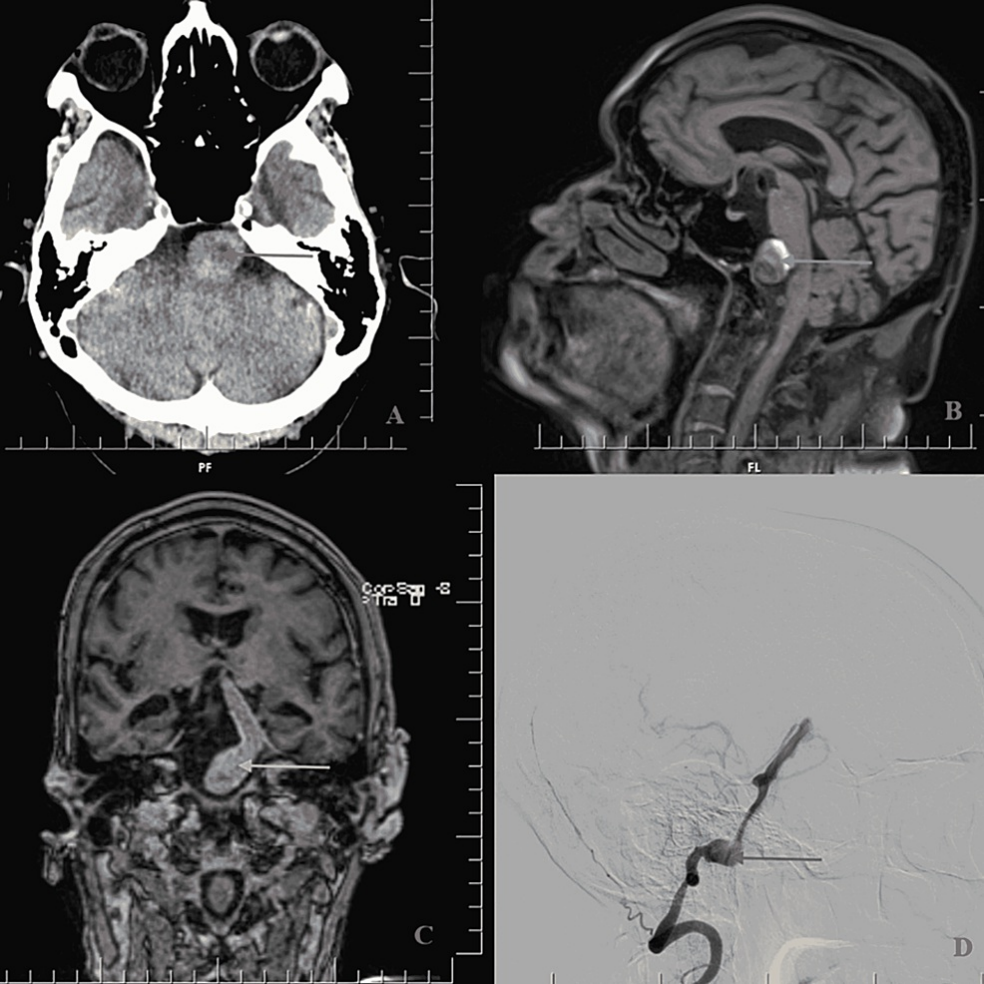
(A) CT brain axial section showing a dilated basilar artery, suggestive of an aneurysm, compressing the left pons (red arrow). (B) MRI brain sagittal section showing a partially thrombosed basilar artery aneurysm (blue arrow). (C) MRI brain coronal section showing a dilated basilar artery, and basilar artery aneurysm (yellow arrow). (D) Cerebral angiogram confirming a fusosaccular aneurysm arising from V3, V4 segments of left vertebral artery and basilar artery (purple arrow). CT - Computed tomography; MRI - Magnetic resonance imaging
